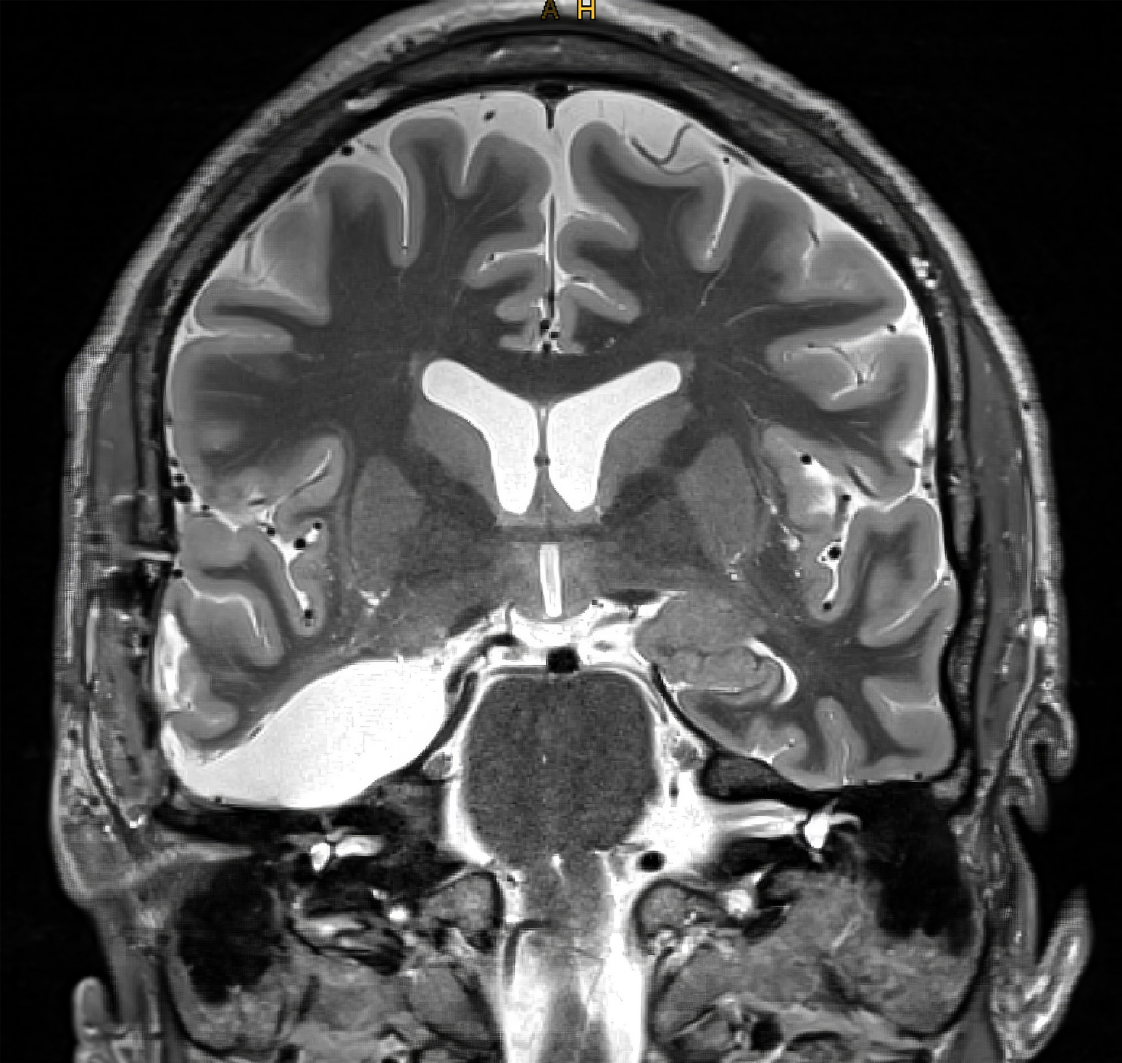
Coronal short‐time inversion recovery sequence of a 3‐Tesla MRI 4 months after surgery/6 weeks after an episode of TGA showing the status after right‐sided selective amygdala‐hippocampectomy.
